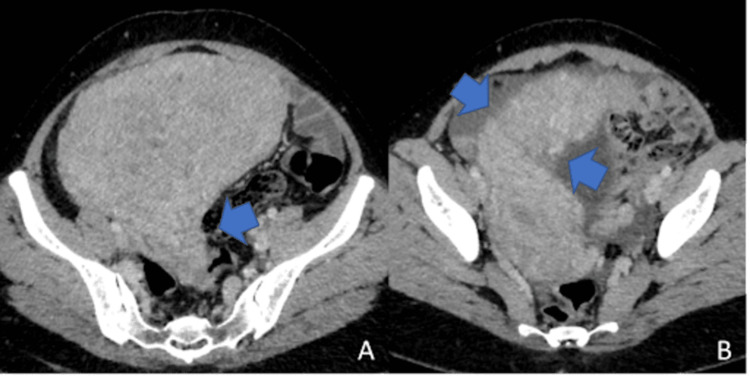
Axial sections of contrast-enhanced CT abdomen-pelvis at different levels (A and B) reveal the “pedicle sign” (blue arrows) connecting the inferior end of the large mass to the right adnexa.
